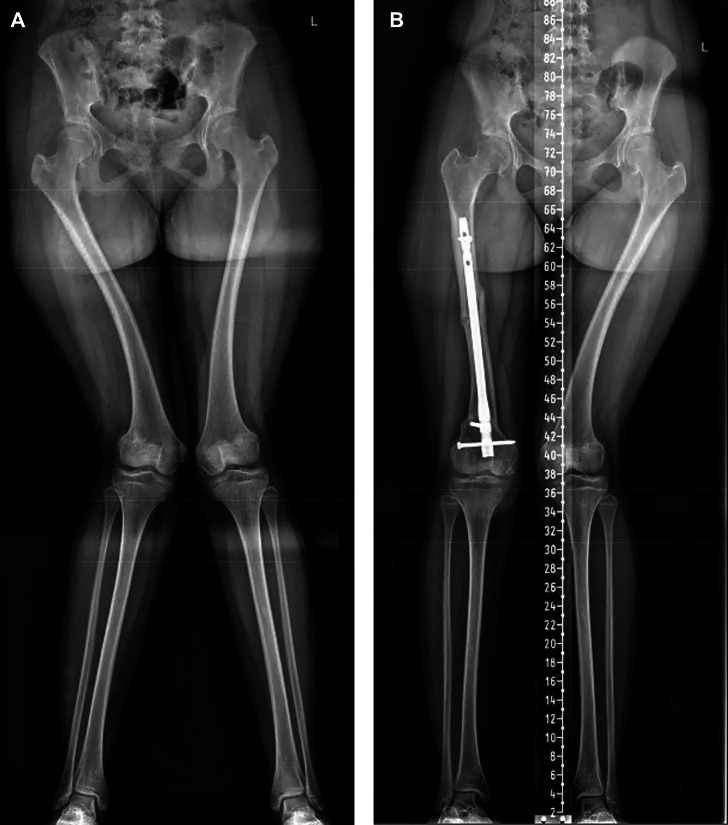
a case of valgus alignment that is femoral in origin; A: pre-op standing full-length radiographs, B: full-lengthen standing radiographs at final fallow-up
